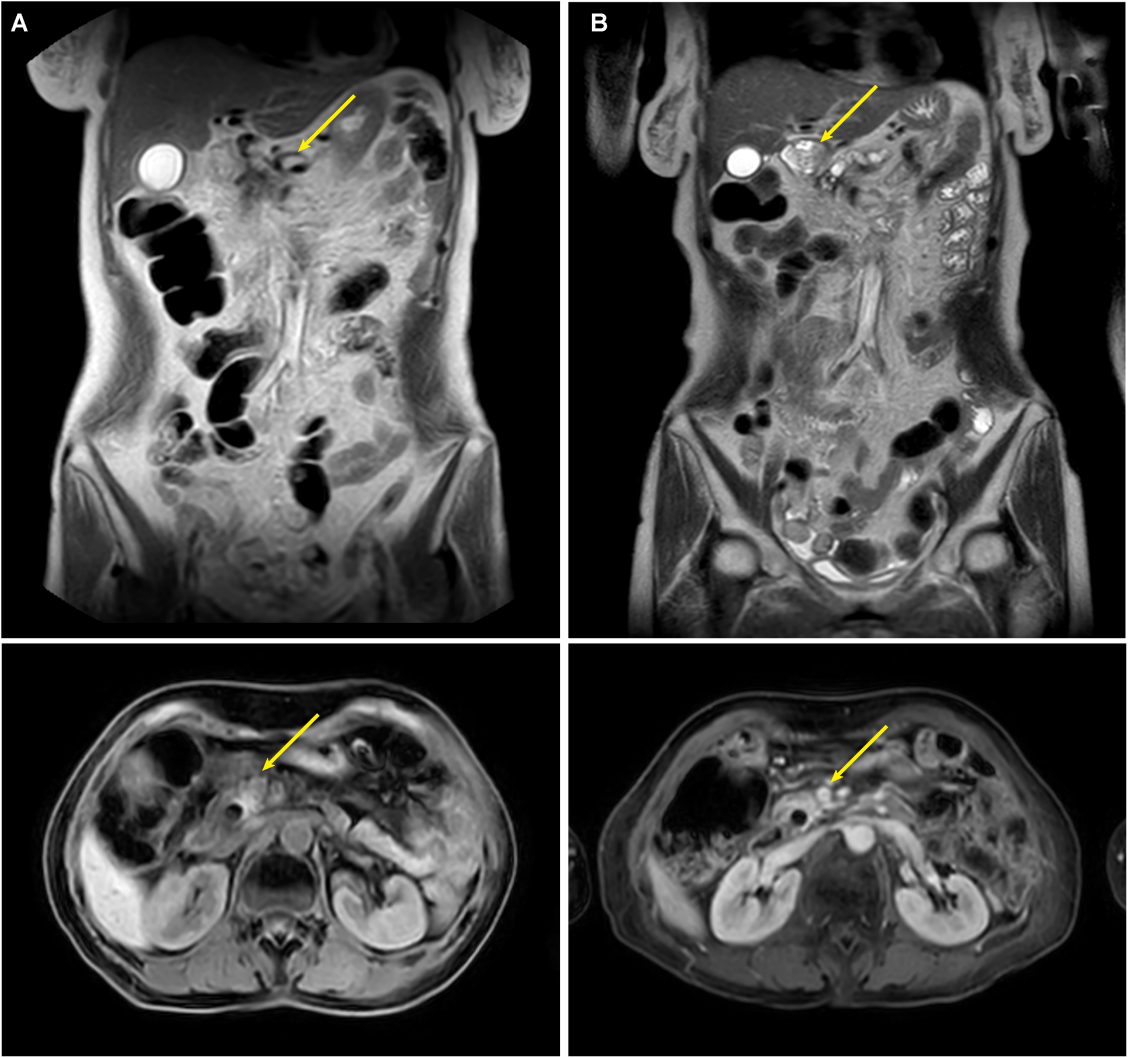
Follow-up MRI at 6 and 12 Months Post-PTCD. MRI demonstrates patent metal stent, with reduction of the pancreatic uncinate mass at 1 year (B) compared to 6 months (A).
